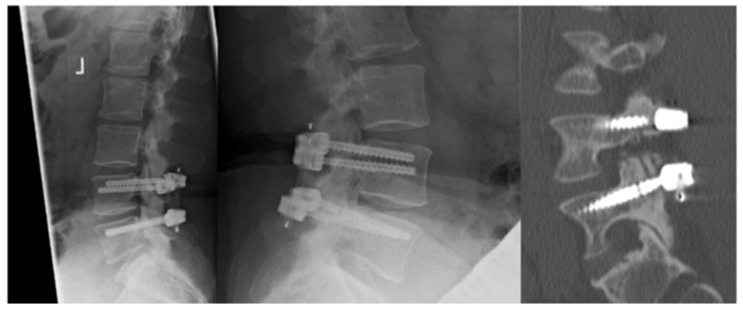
Radiological exam after surgery (left) and after 47 months (middle); CT scan (right).
